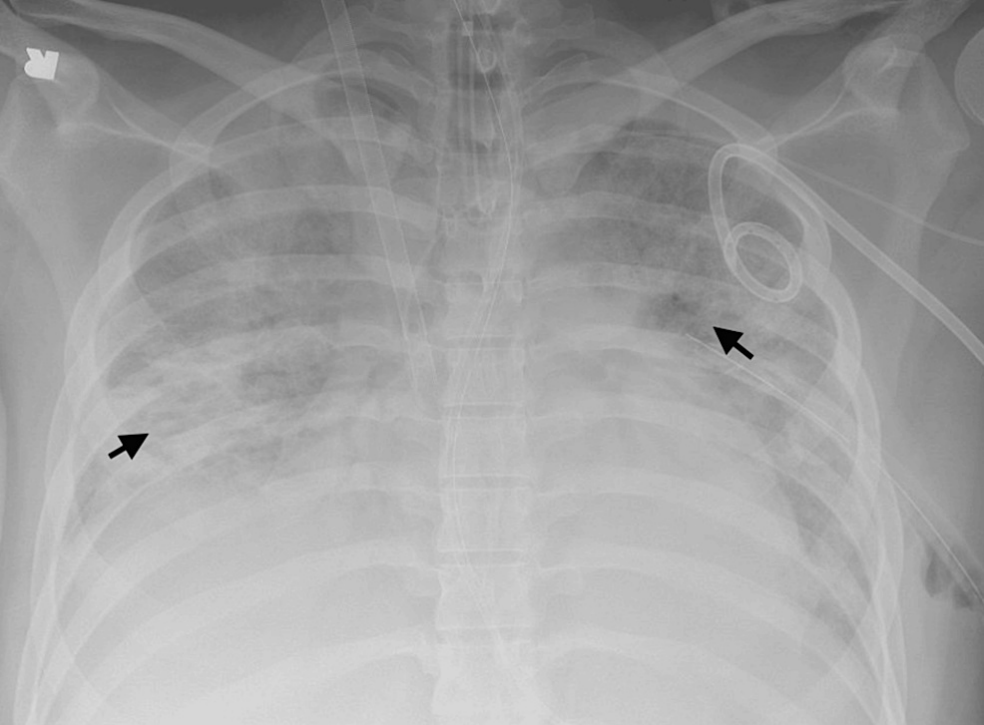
Case 1 chest X-ray Arrows showing bilateral pulmonary infiltrates
